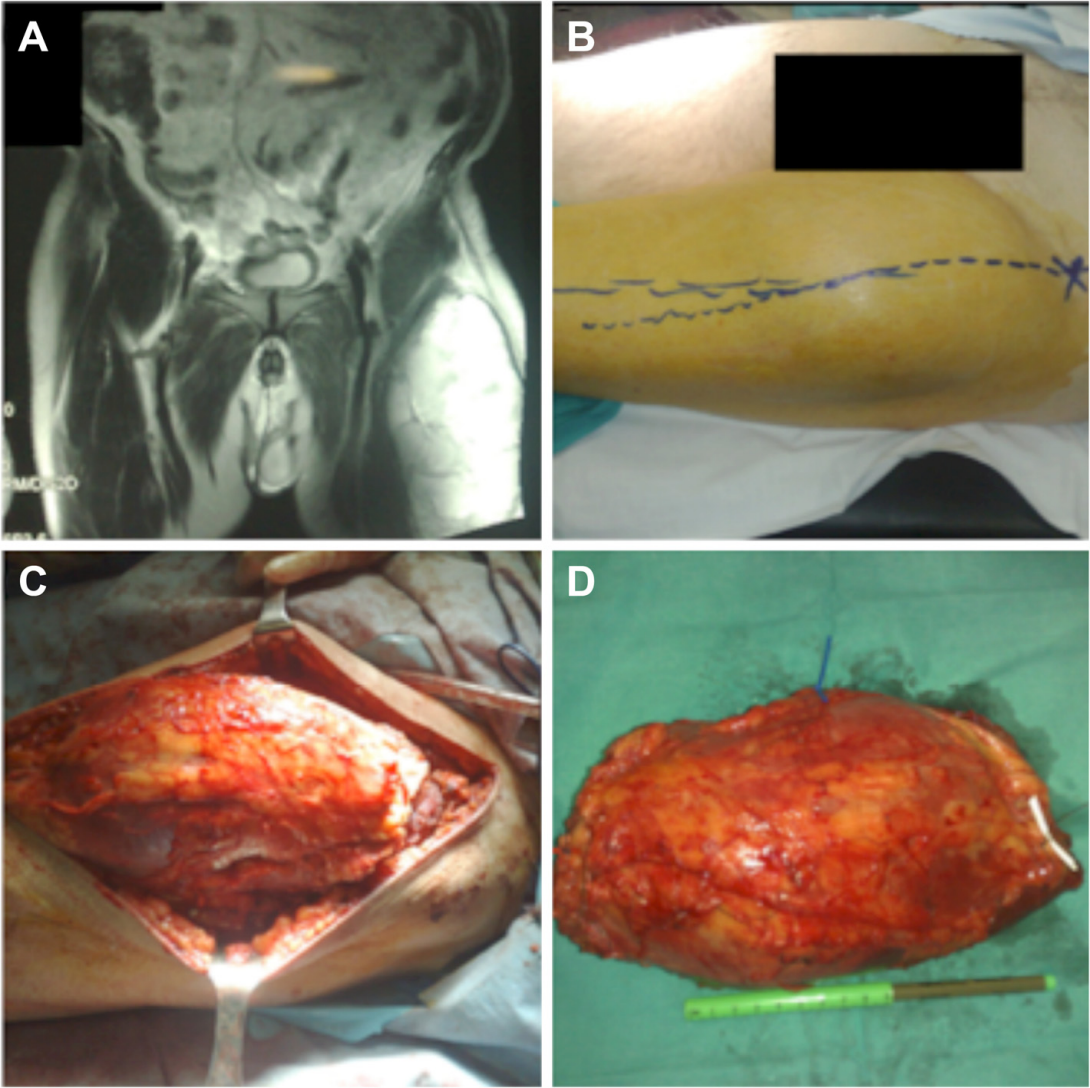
A 69-year-old male with myxoid liposarcoma grade II located in the rectus muscle of the left thigh. a Preoperative MRI, December 2008; b clinical appearance; c intraoperative aspect during surgical resection of the tumour; and d excised tumour part measuring 22 × 15 cm. This patient received adjuvant radiotherapy
